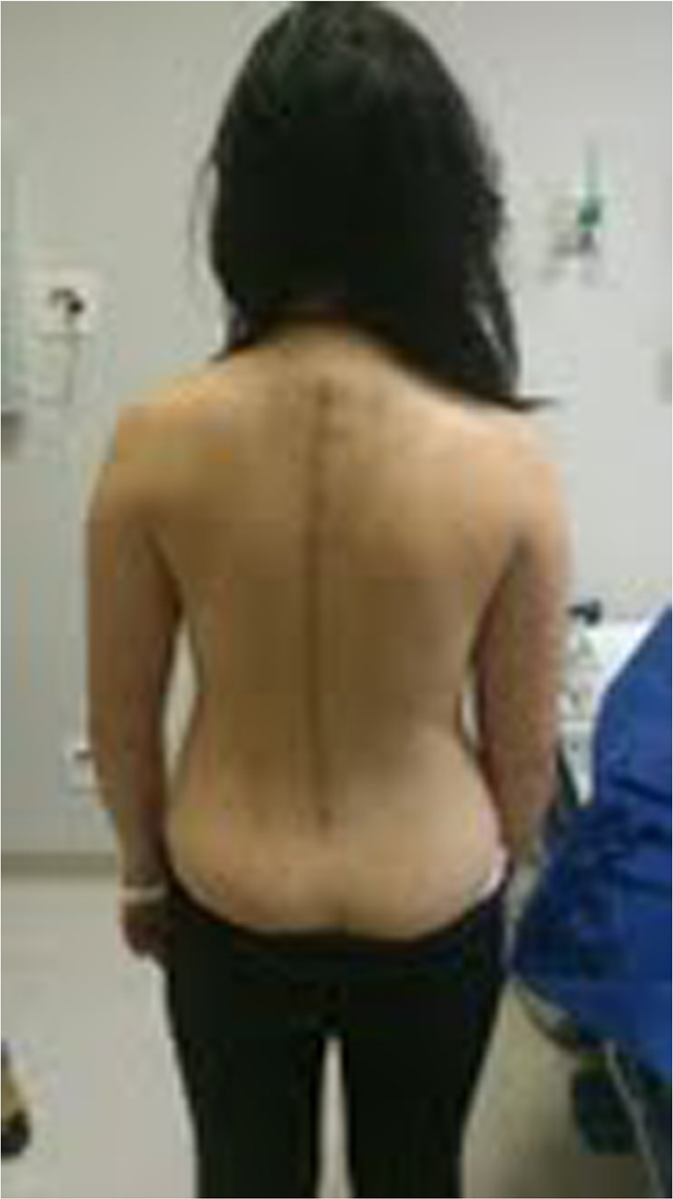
Case 2: The patient after surgery – Radiograph in AP view
